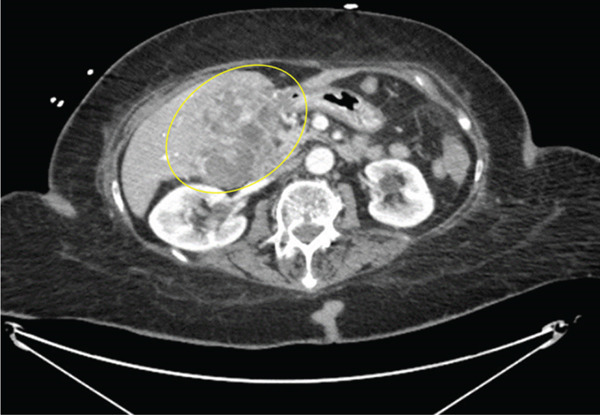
CT abdomen without contrast axial view shows a heterogeneous mass with calcifications 18.2 × 16.7 × 10.7 cm (yellow circle) centered in the gallbladder fossa, demonstrating extension into the liver and transverse colon.
